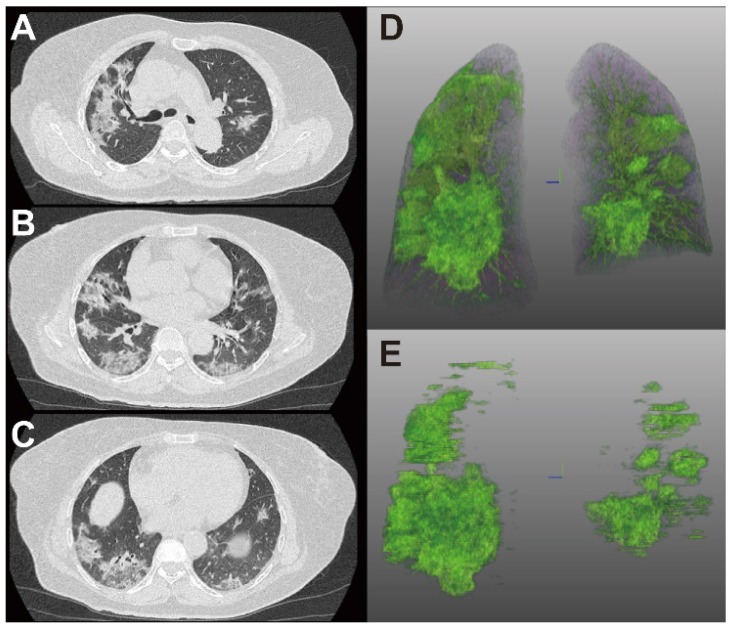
65-year-old woman with coronavirus disease 2019. A-C. Non-contrast CT was performed on day of admission. D. Three-dimensional volume-rendered reconstruction shows the distribution of the opacities. E. Pulmonary opacities segmented by AI system. The patient had history of diabetes and hypertension and showed fever at admission. Patient developed acute respiratory failure at the third day of hospitalization. Lung CT images showed large areas of bilateral consolidation and ground-glass opacities, specifically in the upper lungs.
